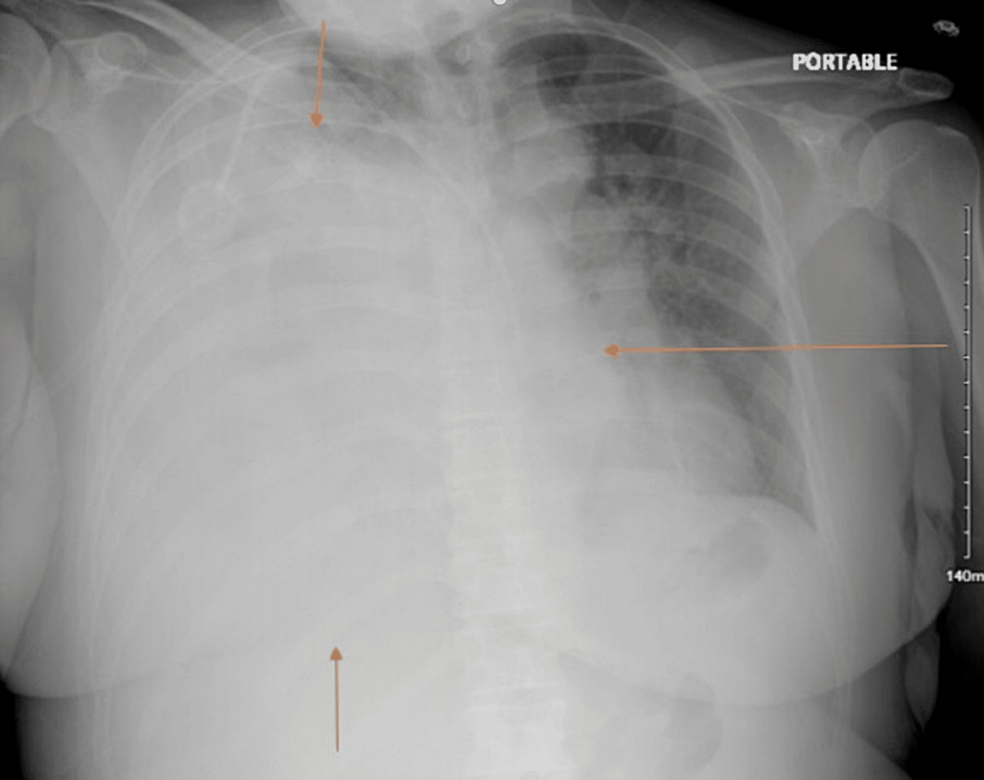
Chest X-ray revealing complete whiteout of the right lung Arrows point towards the area where the mass is occupying lung territory
